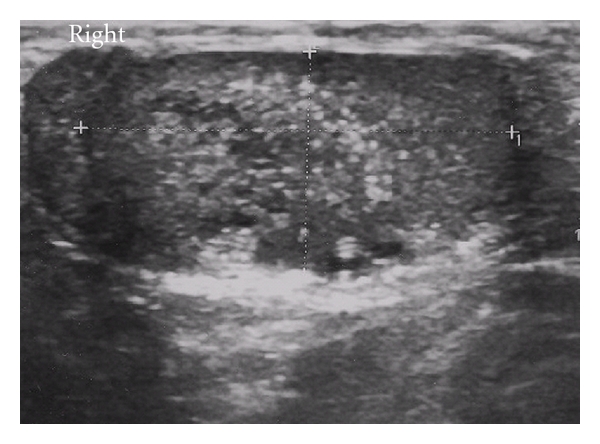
Scrotal ultrasound showing multiple small cystic lesions which comprise about 90% of the right testicular volume and are compressing the normal parenchyma to the periphery.
